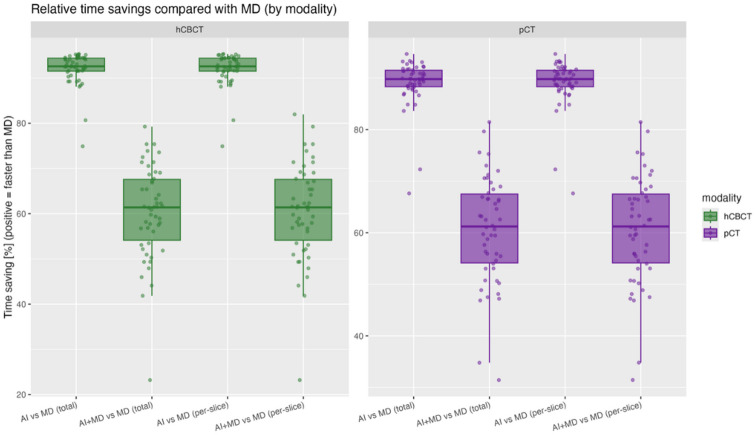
Relative contouring time savings by modality and segmentation mode. Box-and-jitter plots show percent time saving of AI-only autocontouring (AI) and AI-based autocontouring with physician-based validation (AI + MD) compared to manual delineation (MD), displayed for both total time and time per contoured slice, and faceted by modality (planning CT (pCT, purple colours) and HyperSight-CBCT (hCBCT, green colours)). Boxes represent interquartile range (IQR) with median lines; whiskers extend to 1.5×IQR; points are patient-level values (median per patient/mode). Higher positive percentage values indicate faster performance than MD.
